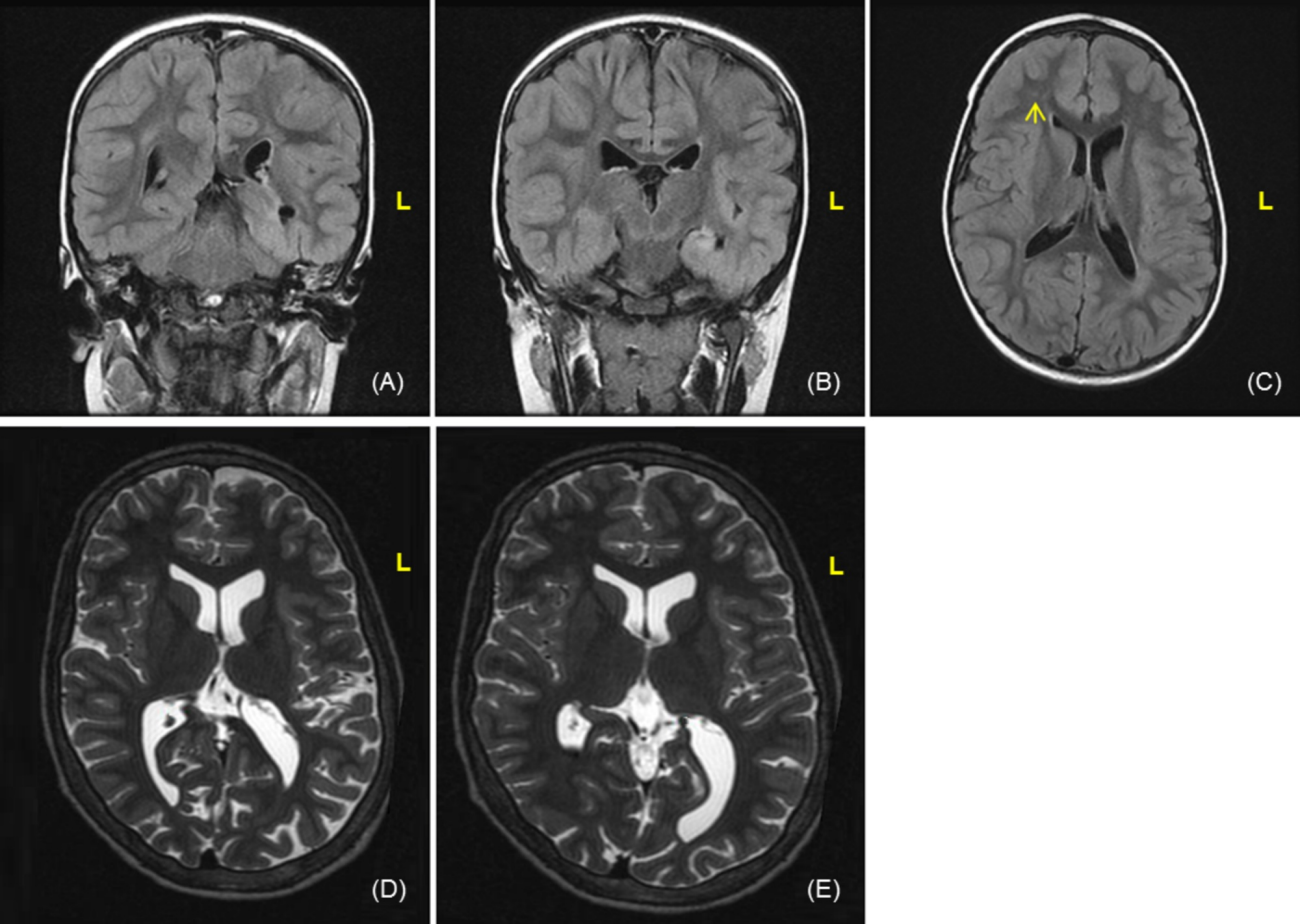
Magnetic resonance imaging of the brain Magnetic resonance imaging of the brain (A-B). There is evidence of abnormal T2 hyperintensity within the left hippocampus and mesial left temporal lobe and mild atrophy of the temporal portion of the left lateral ventricle, which are findings suggestive of mesial temporal sclerosis. In addition, there is evidence of a small focal nonspecific area of T2 hyperintensity seen within the right frontal white matter, which could reflect residua of prior infectious or inflammatory disease or related to seizure activity (yellow arrow) (C). There was no evidence to suggest right-sided mesial temporal sclerosis. There is also congenital enlargement of the occipital horns (D-E).
